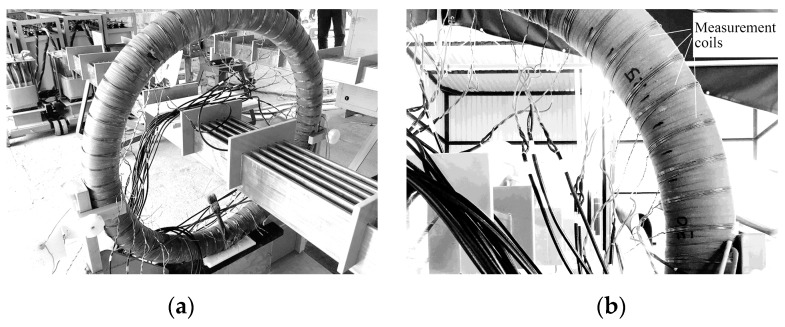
Workplace for the verification experiment of the analytical models. (a) 30 kA CT sample and primary bus-bar. (b) Pre-wound measurement coils.
